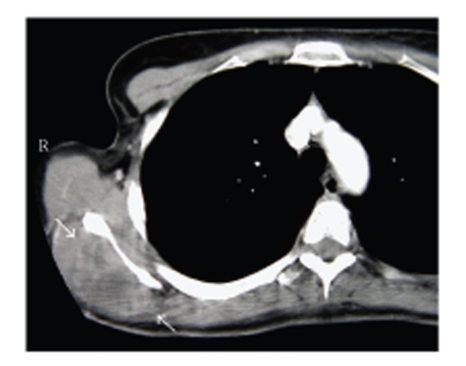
CT scan of the right scapula demonstrating a tumor in the infraspinatus fossa (arrows).
